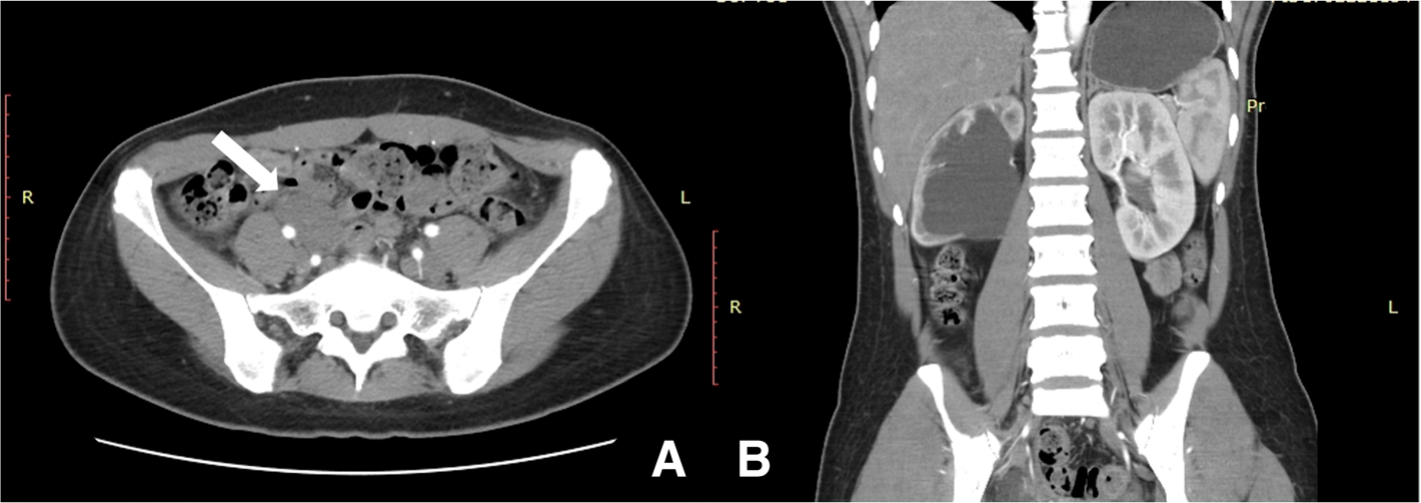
Abdominal CT imageing: pelvic tumors (white arrow) compress right ureter, and cause severe hydronephrosis on the right. a transverse section; b coronal section
